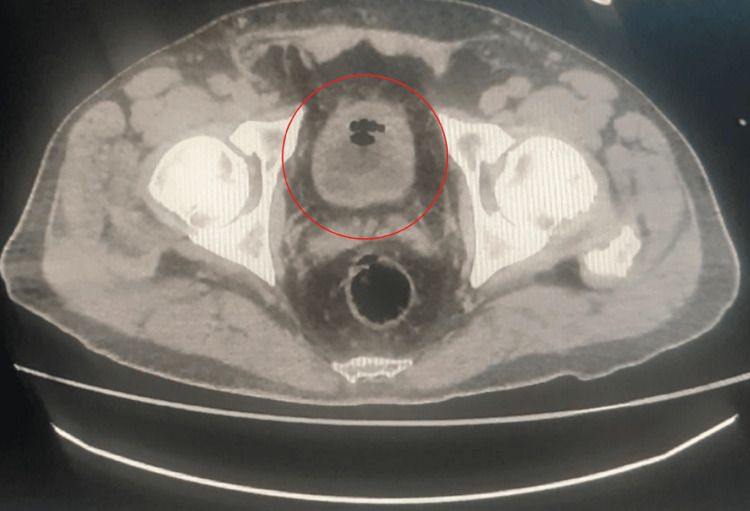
Axial projection of an abdominal CT scan Gas is observed within the lumen of the urinary bladder, consistent with emphysematous cystitis.
